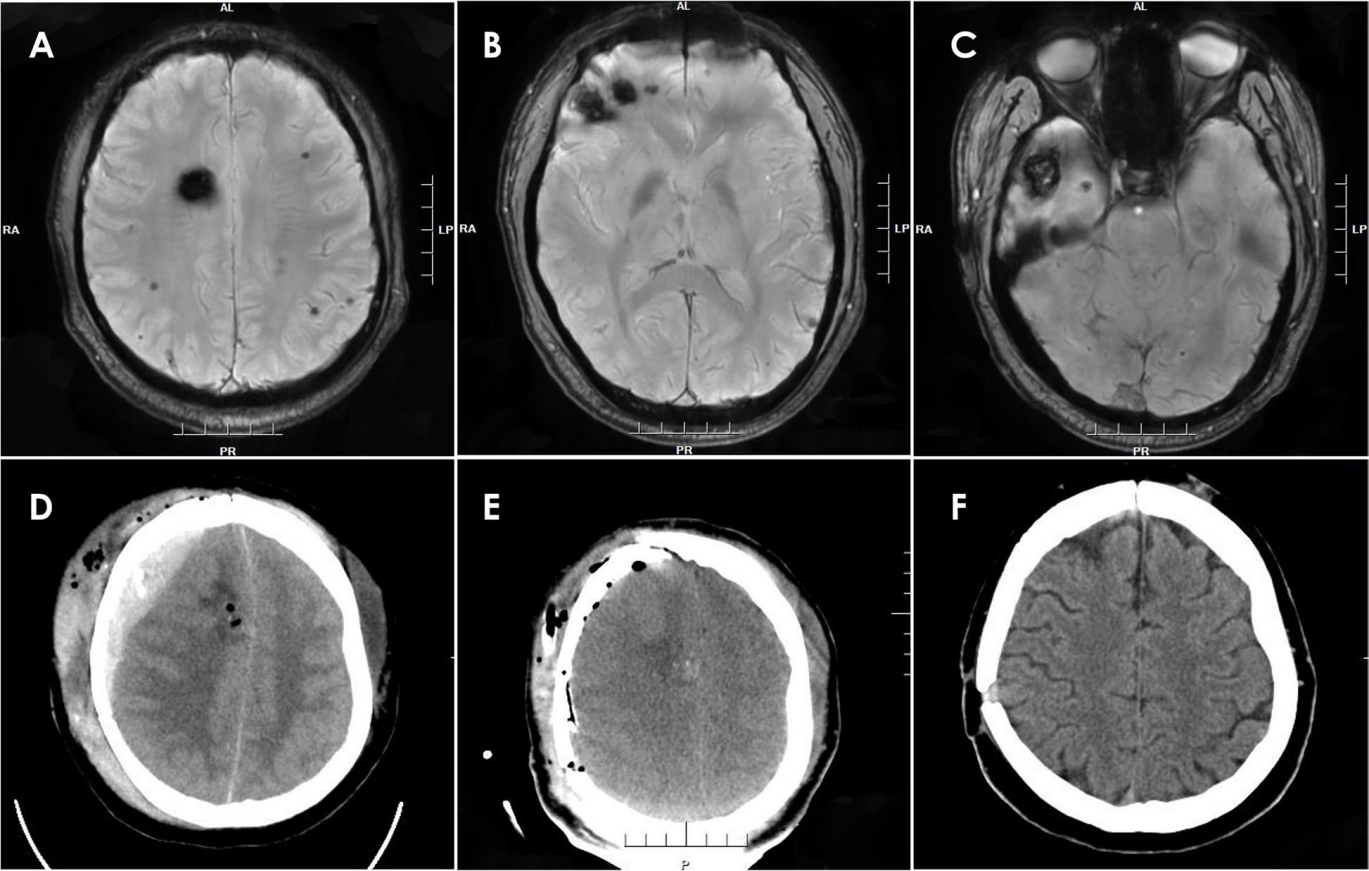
SWI revealing multiple cavernous angiomas (A, B, and C). CT scan demonstrating a type 4 PEDH-epidural hematoma located under the surgical field following tumor resection (D). CT scan showing that the hematoma had been successfully evacuated (E). CT scan obtained 3 months postoperatively (F)
